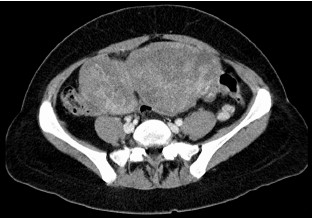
CT scan showing two very large ovarian tumors in the lower abdomen.
